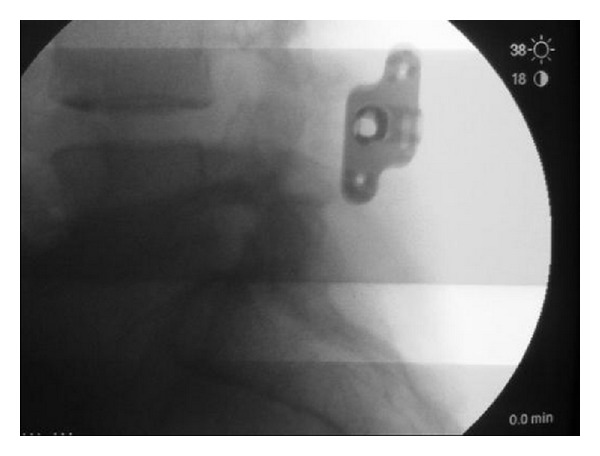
Intraoperative X-ray image of a BacFuse implanted at L4-L5 interspinous level.
